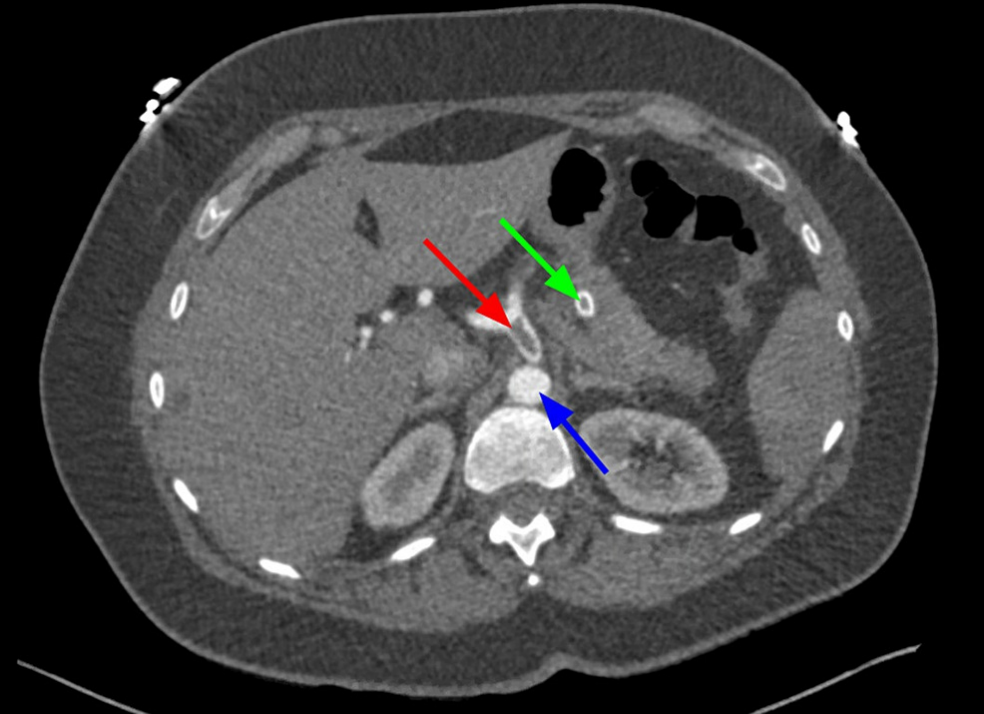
CTA abdomen and pelvis on POD#1 showing thrombus in the celiac artery (red arrow) right after bifurcation from descending aorta (blue arrow) and thrombus in the splenic artery (green arrow). CTA, computed tomography angiography; POD, post-operative day
